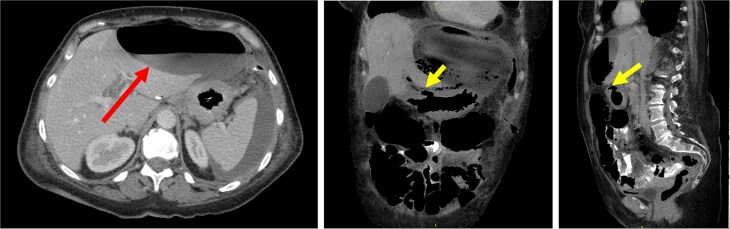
CT images demonstrated a large, thin-walled anterior epigastric collection with an air-fluid level (long/red arrow) measuring 10.8 × 5.8 × 16.4 cm, positioned anterior to the left hepatic lobe and the gastric body. This collection appeared to communicate with the suspected perforation site (short/yellow arrows). Additionally, loculated fluid pockets were observed around the spleen and the left upper quadrant bowel loops, along with interspersed free fluid and gas locules likely leaking from the stomach.
